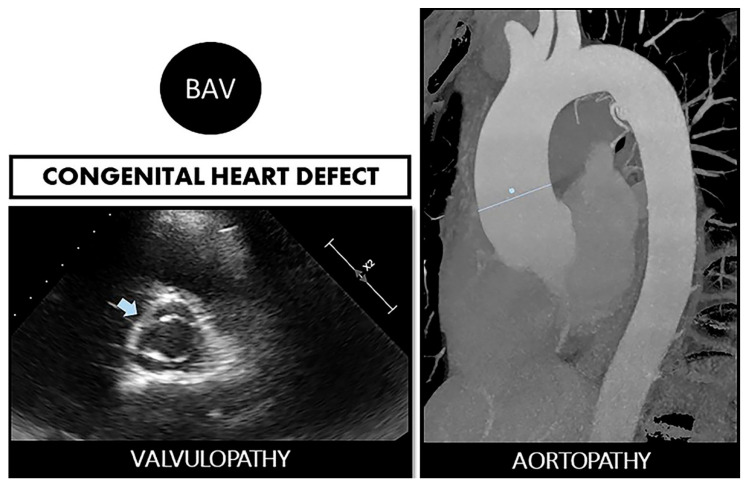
Bicuspid aortopathy is a double road. The congenital defect can develop in the person who suffers from both valvulopathy (stenosis or regurgitation) and aortopathy (aneurysms and risk of spontaneous dissection). On the left, echocardiographic image of BAV (arrow). On the right, a resonance image of dilated ascending aorta (marked straight line).
